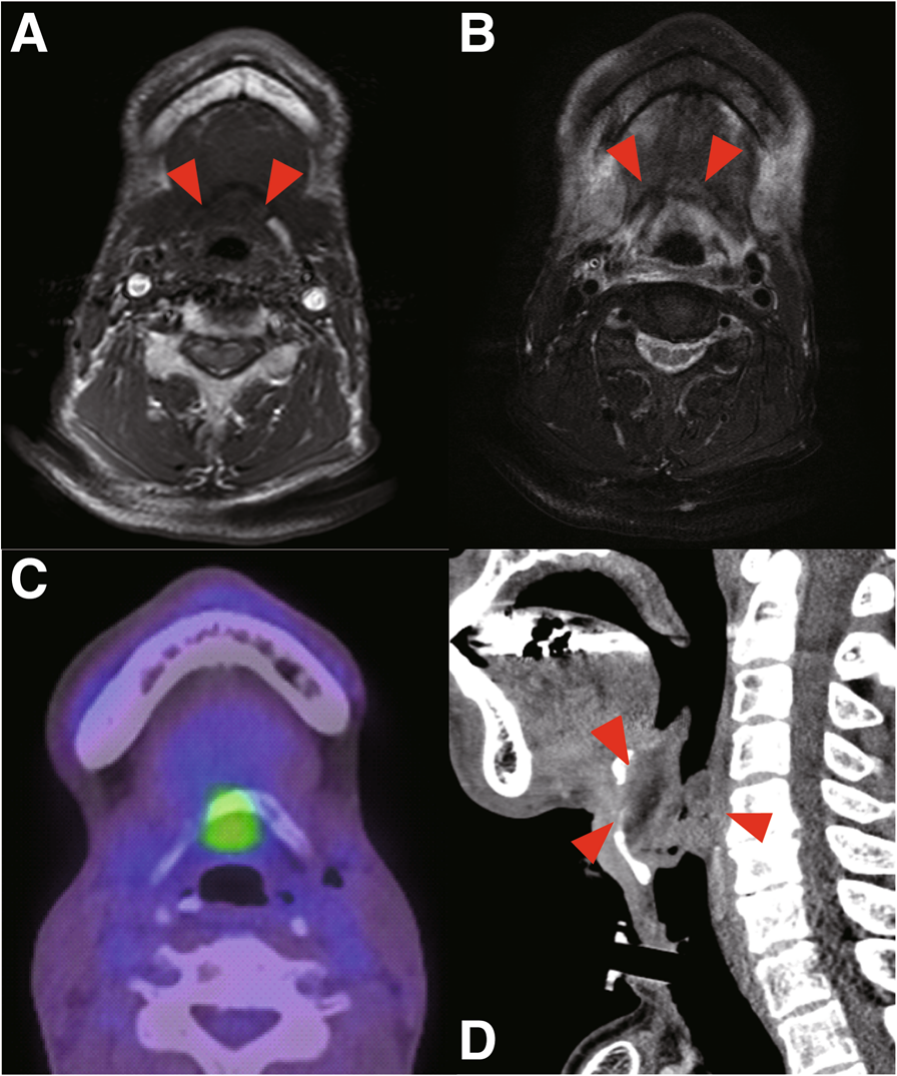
a Signal intensity decreased in the hyoid on T1WI, b Mixed high and low signal intensity on T2WI The *arrows* indicate the ORN of hyoid bone. c PET-CT shows focal high FDG activity in the hyoid and larynx. d Sagittal view of CT scan in the larynx. The *red arrows* indicated edematous swelling of laryngeal mucosa and stenosis
